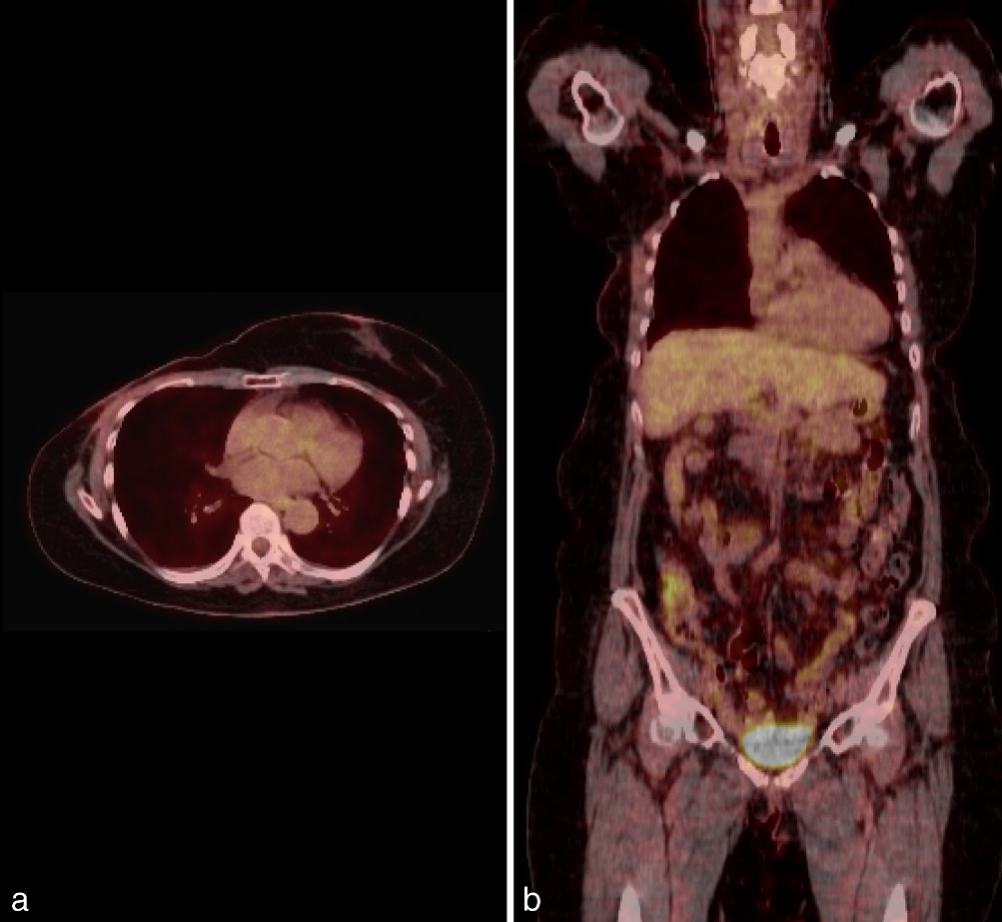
(a) Axial and (b) coronal views of 18F-fludeoxyglucose PET-CT scan performed 12 months postoperatively showing complete resolution of previous ^18^F-fludeoxyglucose uptake with no evidence of ^18^F-fludeoxyglucose-avid disease.
